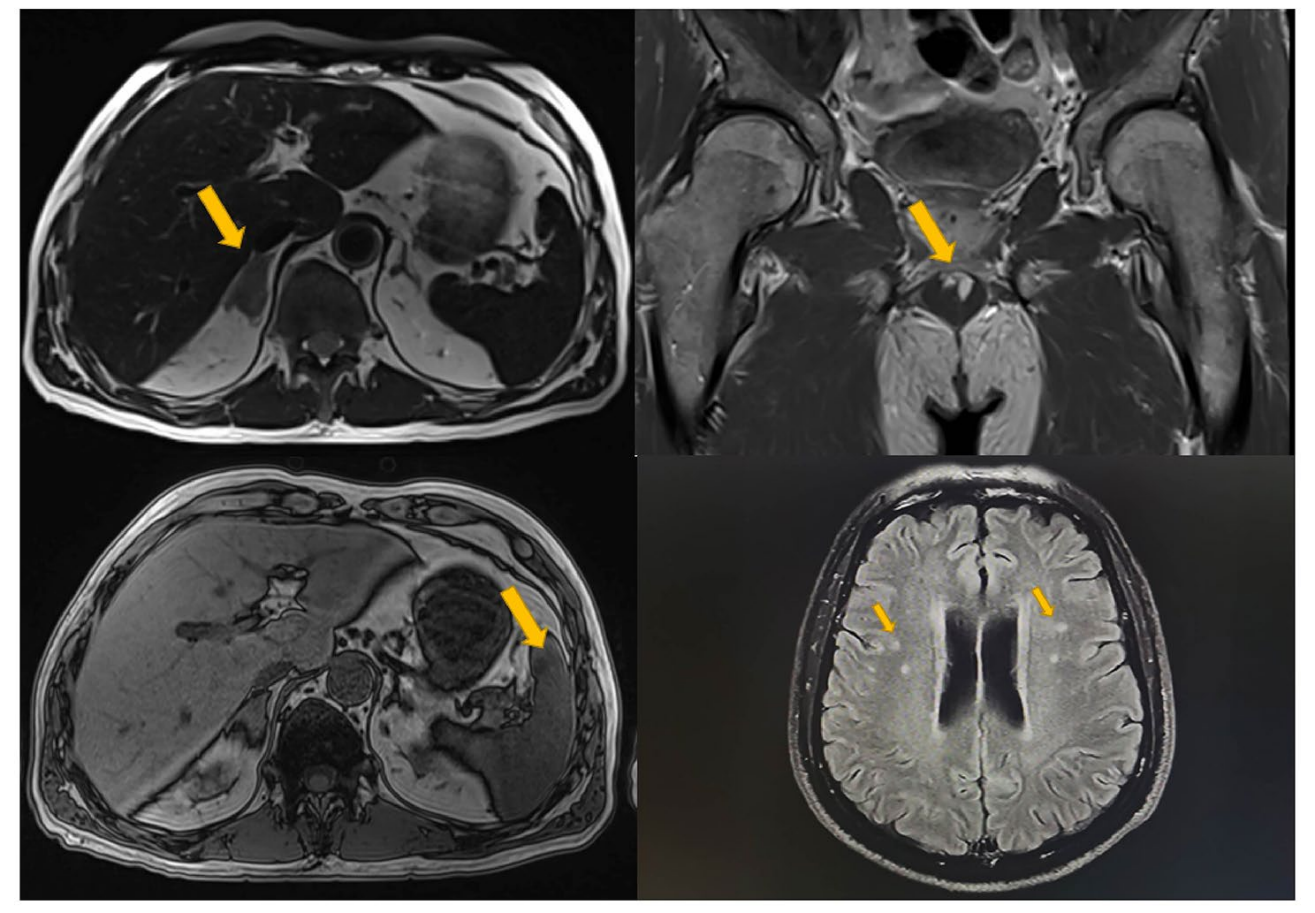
Multiple nodules found in MRI(upper left: adrenal gland, left bottom: spleen, upper right: prostate, right bottom: brain.)
